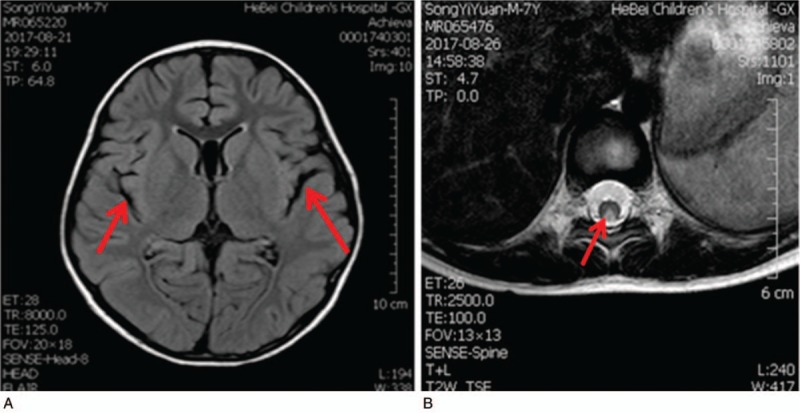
MRI findings of the patient. (A) The enlargement of the cerebral groove in the skull MRI. (B) The axial position of the thoracic segment shows the hyperintense T2W1 signals in the posterior columns, presenting an inverted “V” sign. MRI = magnetic resonance imaging. T2W1 = T2 weighted image.
